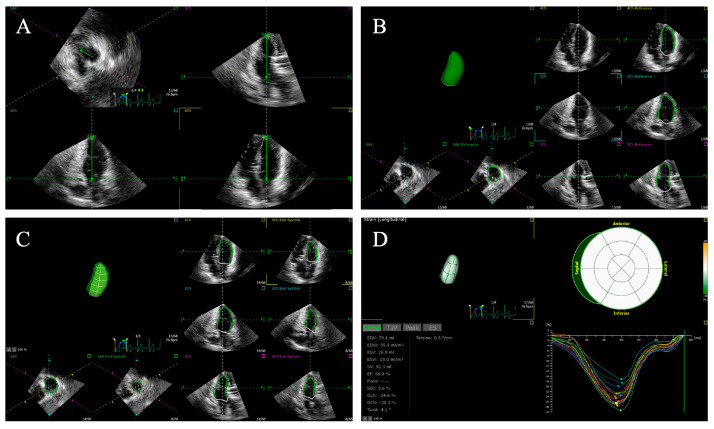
Left ventricular global longitudinal strain using three-dimensional speckle tracking echocardiography. (A) Setting reference points; (B,C) left ventricular endocardial border tracking; (D) left ventricular global longitudinal strain is automatically generated.
